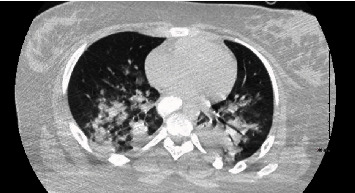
CT of the lungs demonstrates bilateral mid- and lower lung patchy consolidations and ground-glass opacities extending into the bilateral lung bases. Bilateral air bronchograms are noted.
